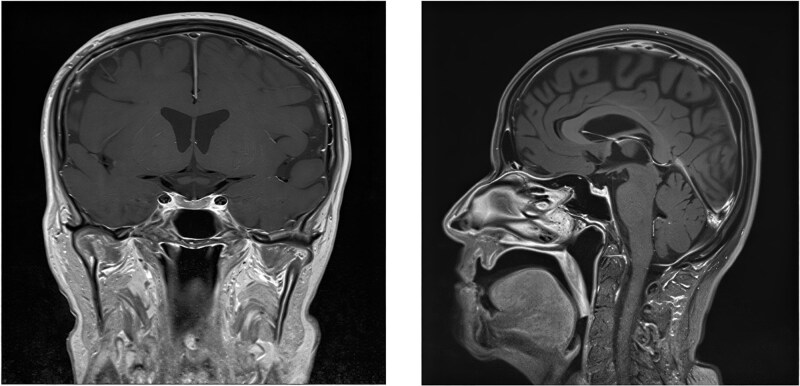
Post-contrast T2-weighted magnetic resonance imaging (MRI) demonstrates normal pituitary.
